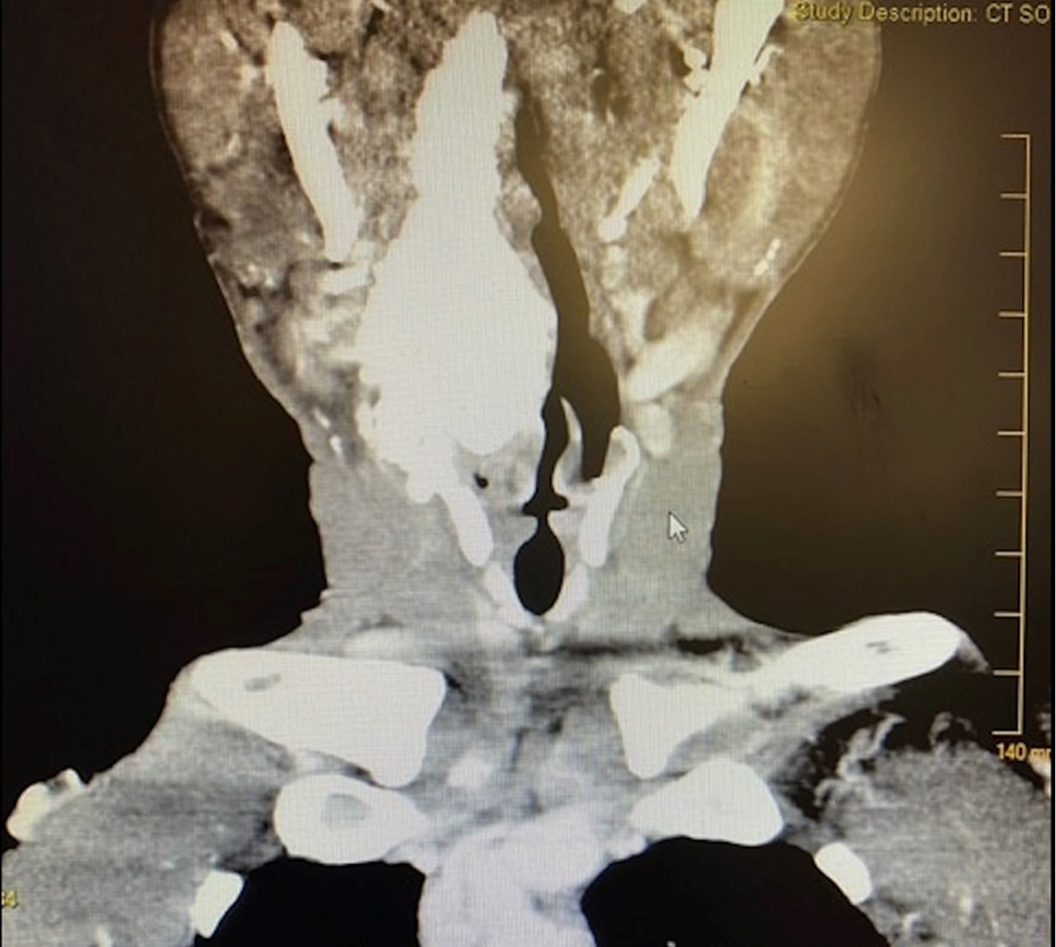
Coronal view, CT soft tissue neck. An amorphous calcified soft tissue mass lesion
